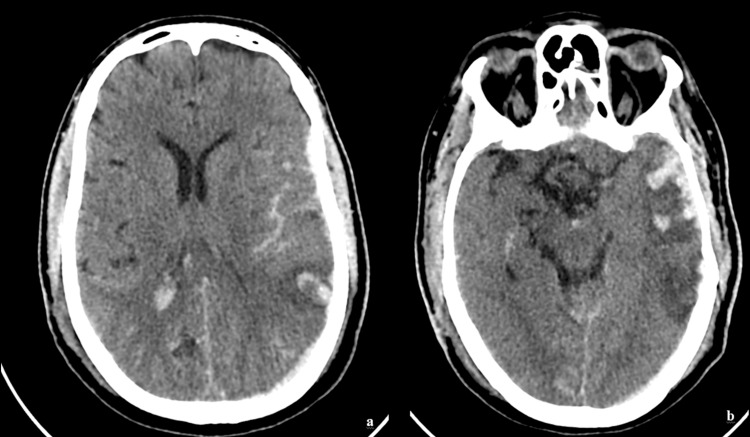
(a and b) A 55-year-old male who was involved in a road traffic accident and sustained a head injury presented with a Glasgow Coma Scale (GCS) of 7. The initial computed tomography (CT) scan of the brain (axial section, 1 mm slice thickness, standard mediastinum algorithm with a window level of 10 Hounsfield units {HU} and a width of 300 HU) shows a Rotterdam computed tomography score (RCTS) of 5, indicating severe traumatic brain injury (TBI).
